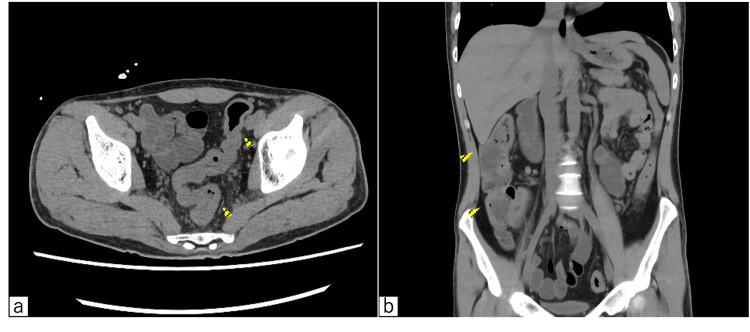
The plane CT scan at the time of hospitalization (a) The axial view of the pelvis The marker indicates thickening of the rectal wall. (b) The colonal view The marker indicates thickening of the right colonic wall. Diffuse thickening of the colonic wall extending from the rectum to the cecum, consistent with pancolitis-type UC. UC: Ulcerative colitis
